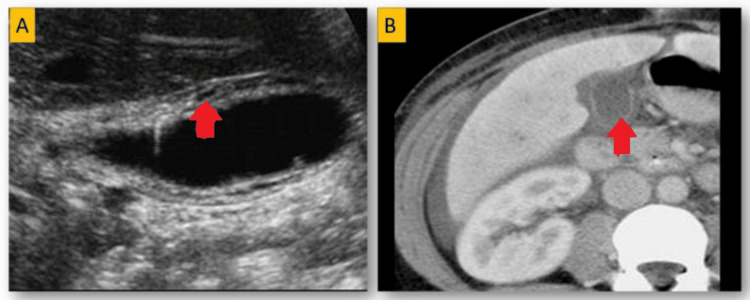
Radiology pictures of IGBC A: Digital ultrasonography picture of IGBC showing thickened gallbladder wall. B: CT image of IGBC showing thickened gallbladder wall. Abbrevations: IGBC=incidental gallbladder carcinoma
